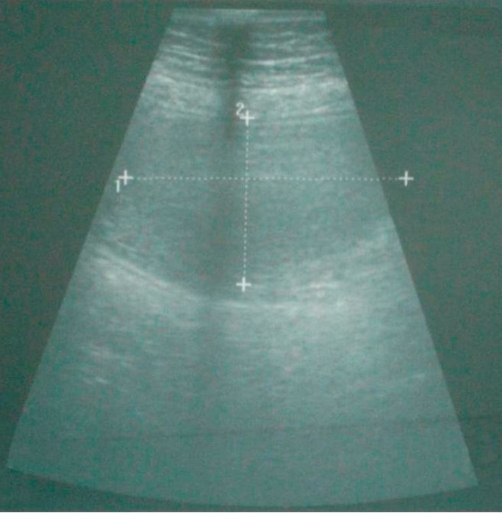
Ultrasound examination: a transverse section of an ileal loop shows an echogenic 7-cm intraluminal mass.
